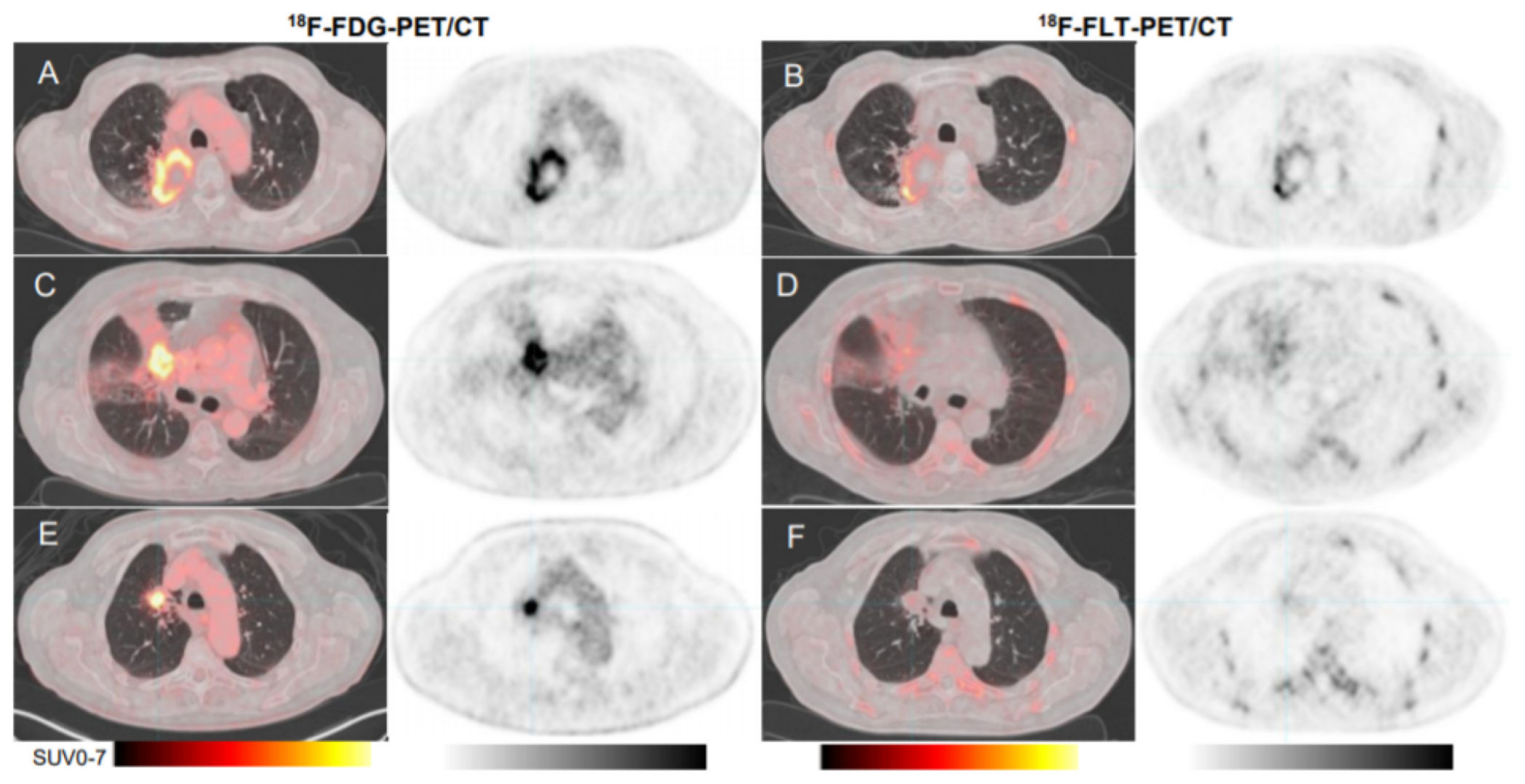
FDG-PET/CT and FLT-PET/CT in three representative patients with suspected relapse after conventional fractionated radiotherapy (cRT) of a lung cancer. A-B: Relapse 19 months after end of cRT detected by FDG-PET/CT (A) and FLT-PET/CT (B). C-D: No relapse 4 months after end of cRT. FDG-PET/CT (C) was false positive. FLT-PET/CT (D) was true negative. E-F: Relapse 15 months after end of cRT. FDG-PET/CT (E) was true positive. FLT-PET/CT (F) was false negative. The relapse was located in lung tissue as confirmed by biopsy, not in lymph node as it may appear on these examinations.
